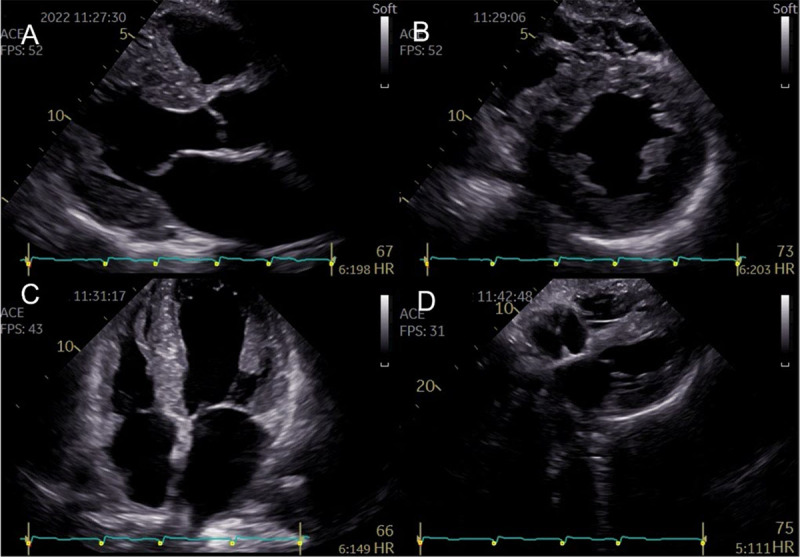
Representative two-dimensional echocardiographic findings of cardiac amyloidosis in a patient with ATTR-CM. (A) Parasternal longitudinal view (B) Short axis view (C) Apical 4-chamber view (D) Subcostal view Concentric left ventricular and right ventricle free wall hypertrophy, thickened interatrial septum, and atrioventricular valves. (Images courtesy of Centro Hospitalar Universitário Lisboa Norte, Lisboa, Portugal).
